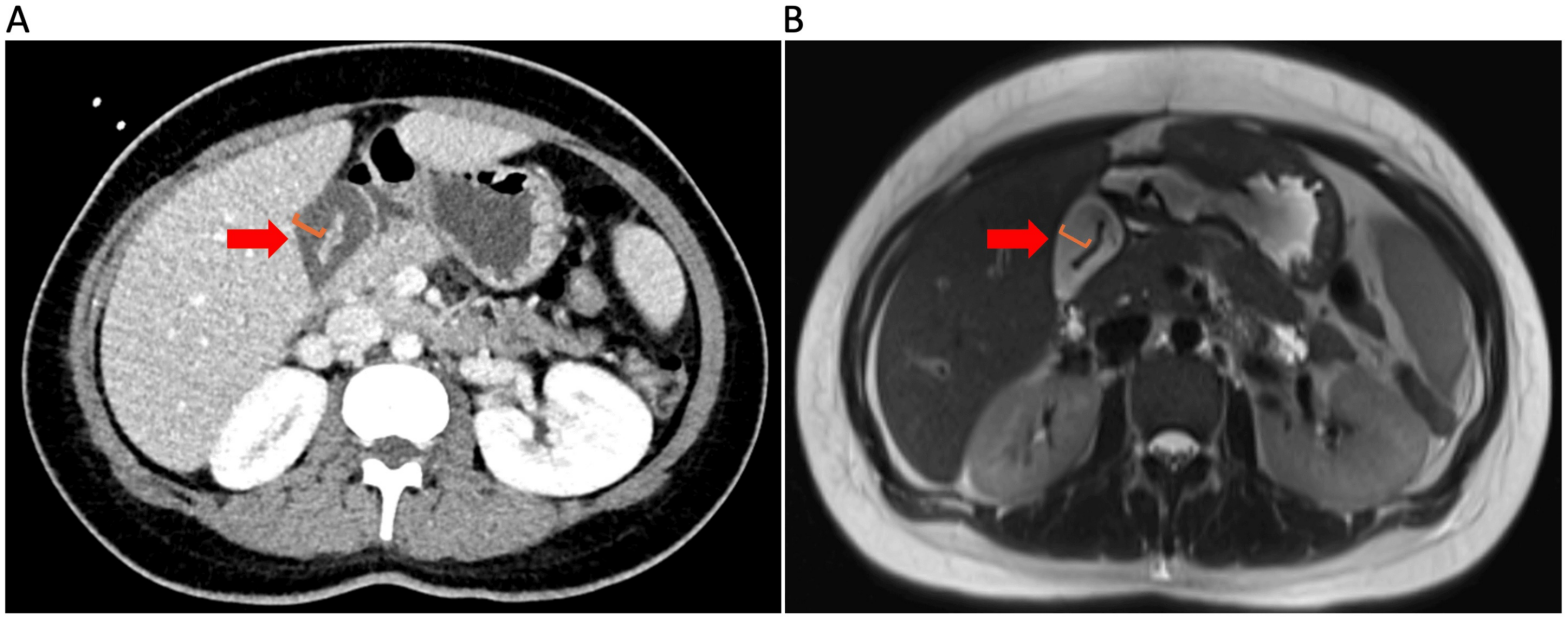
The axial images of a thickened gallbladder on imaging. Arrows in red indicate the gallbladder, which has a thickened wall and a collapsed lumen. Scales in orange show the thickened gallbladder wall. These radiological characteristics were demonstrated on CT (A) and MRCP (B). CT, computed tomography; MRCP, magnetic resonance cholangiopancreatography
